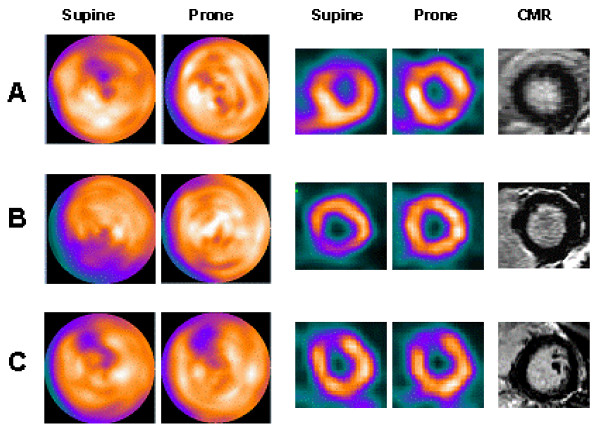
Comparison of supine and prone SPECT imaging with corresponding CMR imaging. The 2 left columns show SPECT bull's-eye plots; the 2 middle columns representative SPECT short-axis images; and the right column the corresponding CMR images. The prone polar maps represent raw data and are not normalized to a normal database. A: a female patient with an apparent anterior-wall defect in the supine position that disappears in the prone position, CMR was normal (no signs of infarction). B: a male patient with an apparent inferior-wall defect in the supine position that disappears in the prone position, corresponding with a normal CMR. C: a defect that persists from supine to prone position in a female patient. This patient had normal CMR results. A and B are examples of the prone images correctly excluding myocardial infarction, whereas C is an example of a prone image that failed to correct for an attenuation artifact.
